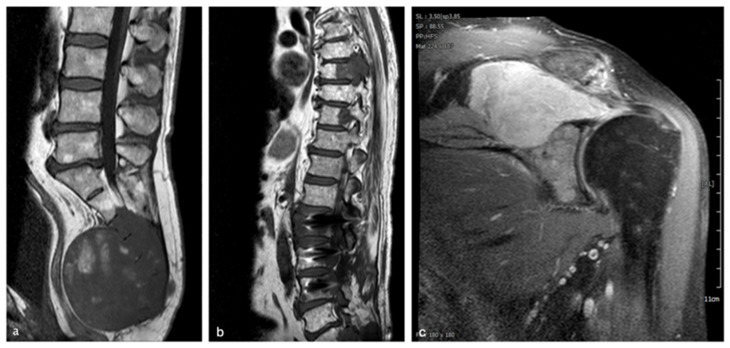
An MRI of a 70-year-old patient with sacrococcygeal chordoma. The tumor shows low signal intensity on T1-weighted images (a), with small foci of hyperintensity indicative of intratumoral hemorrhage. (b) An MRI of the same patient 7 years later, after he presented with an acute transverse lesion and received immediate radiation therapy. The sagittal T1 imaging plane shows two intraspinal lesions originating from TH6-TH8 with absolute spinal canal stenosis and possible infiltration of the myelon, as well as an increase in the size of a previously known space-occupying lesion in the lumbar spine region. The T1/TSE coronal fat-suppressed imaging plane (c) with intravenous contrast shows a metastasis in the processus coracoideus of the left shoulder with destruction of the glenoid; this was first diagnosed three years earlier but was left untreated because the patient had a poor cardiovascular condition.
